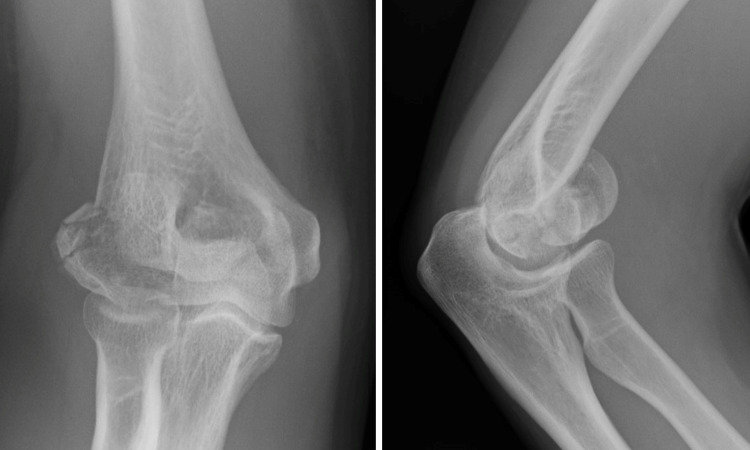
A preoperative radiograph of case 1 showing a fracture from the lateral distal humerus to the trochlea, with a positive double arc sign on the lateral view.
